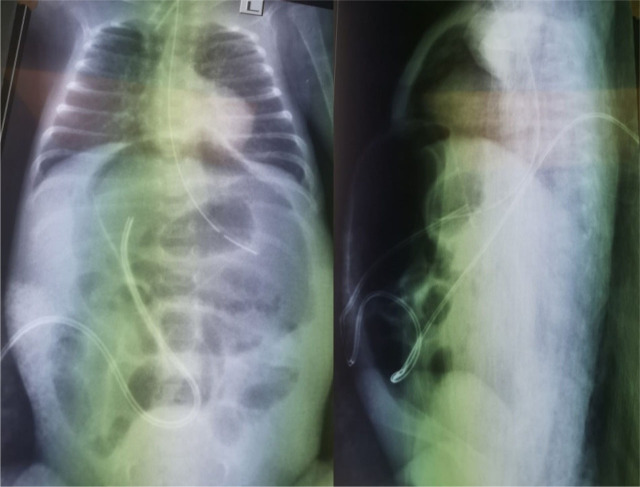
Abdominal X-ray: (A) Anterior-posterior view: thickened, dilated bowel loops marked with arrow (B) Lateral decubitus view: pneumoperitoneum – marked with arrow
